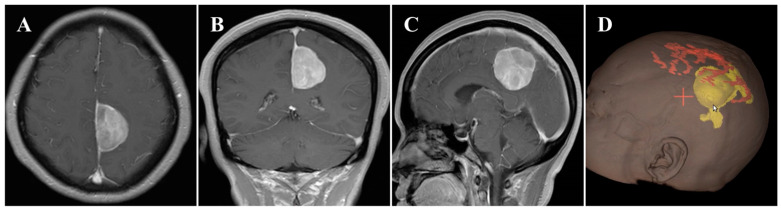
Preoperative imaging and intraoperative navigation showed a middle-third falcine meningioma. (A–C) Contrast-enhanced MRI axial (A), coronal (B), and sagittal (C) images. (D) Neuronavigation (3D image) demonstrated two drainage veins above the tumor (white arrow).
